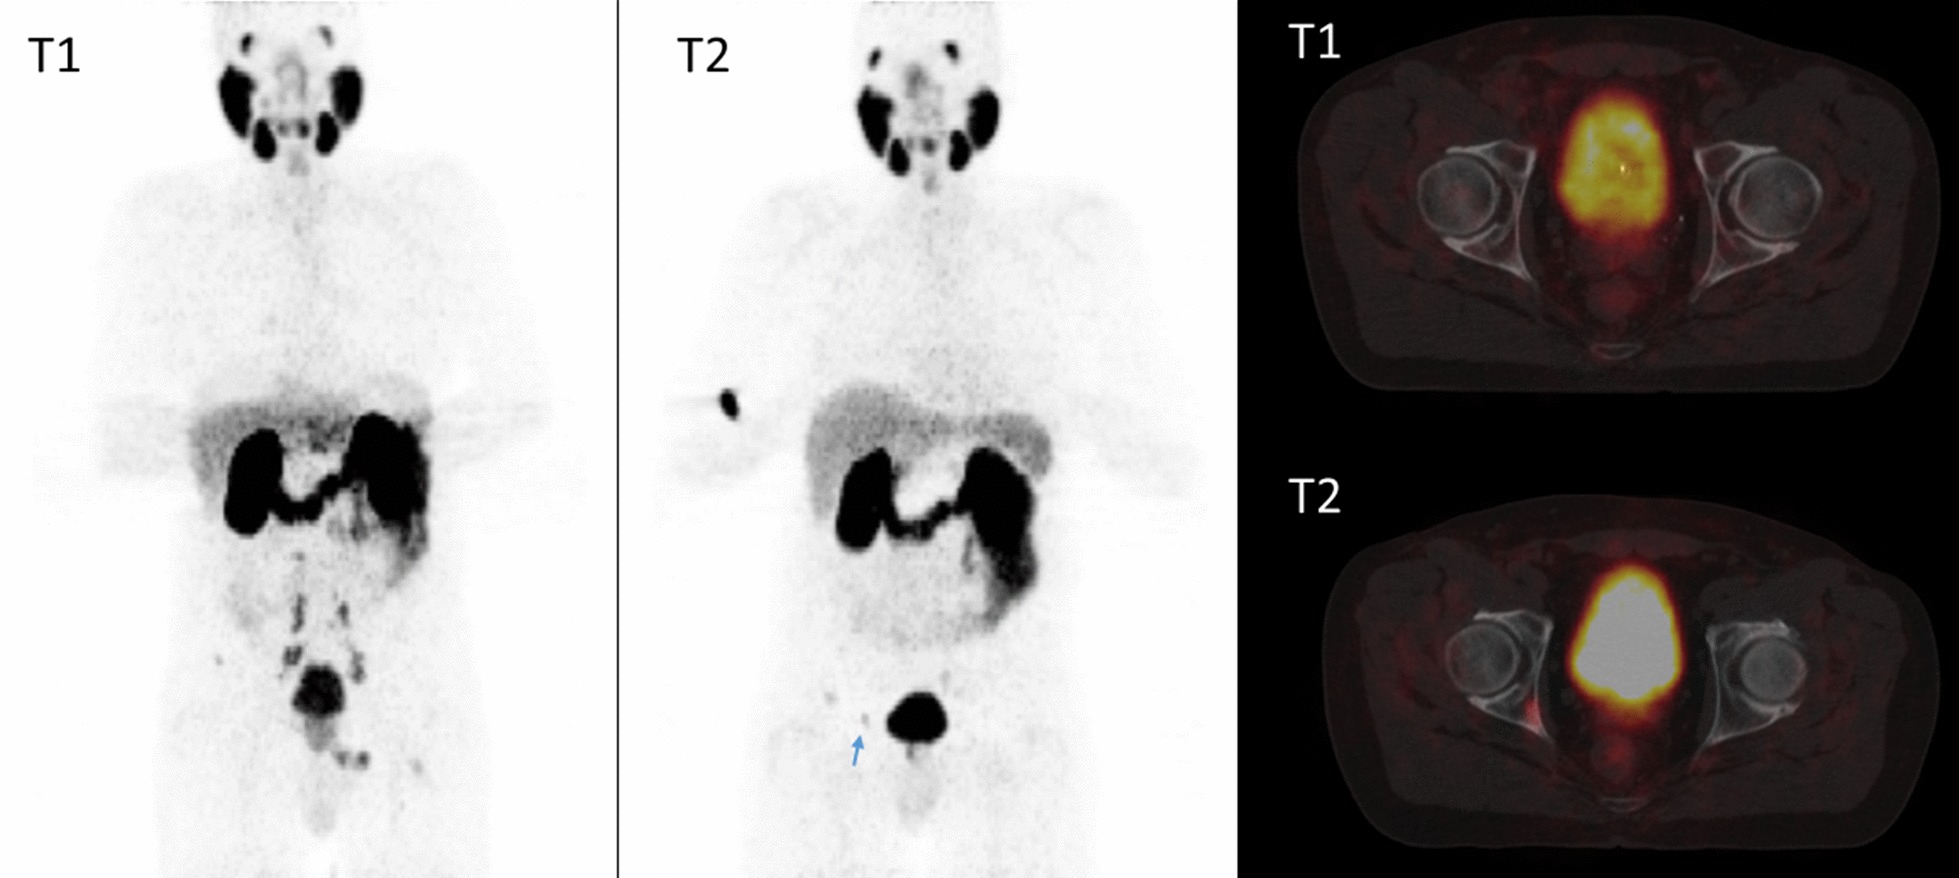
MIPs and fused PET/MRI at T1 and PET/CT at T2. There is no pathological PSMA expression in either the prostate or the metastatic sites (arrows in the fused image at T1) at T2
